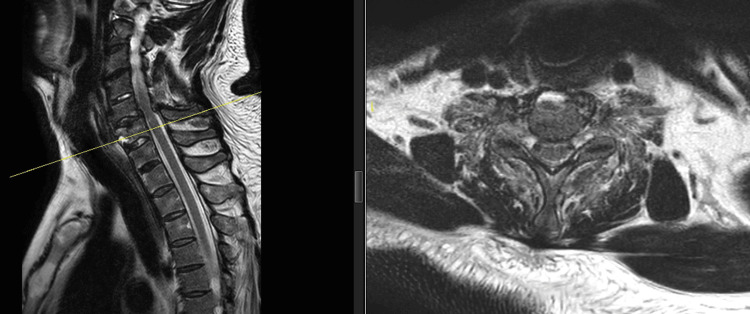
T2-weighted image shows C6 body fracture with spinal stenosis and cord edema
